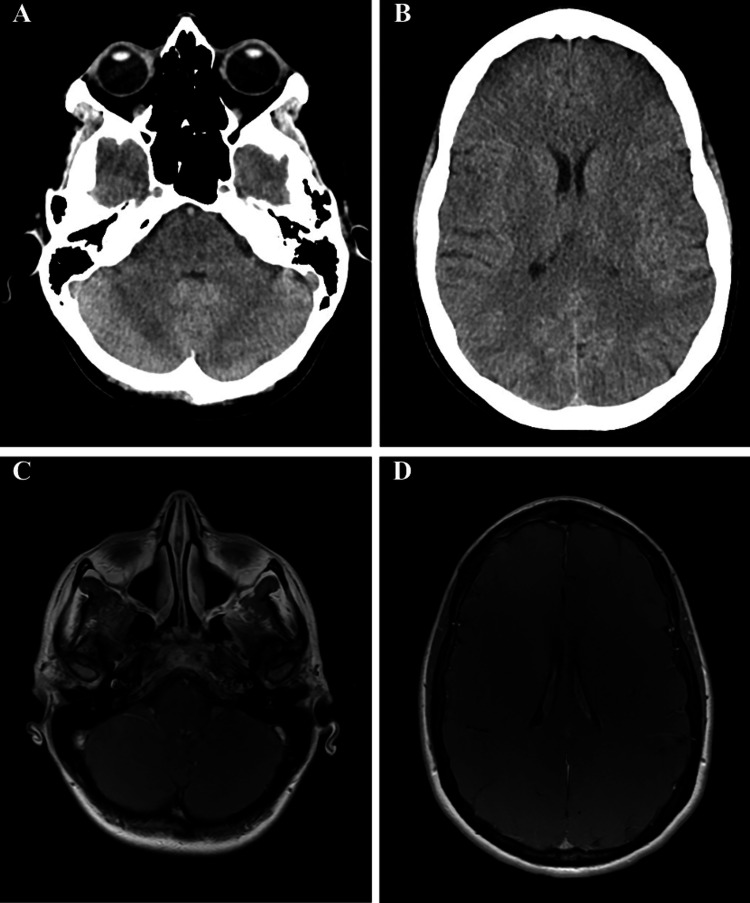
CT and MRI of the brain with contrast. Panels A and B show a non-contrast CT assessing for calcifications, while panels C and D show a contrast-enhanced MRI evaluating for abnormal enhancement. No calcifications or abnormal enhancement were observed.
